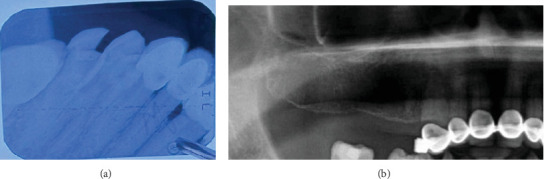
(a) Periapical x-ray before the extraction of #14. (b) X-ray after the extraction of #14.
